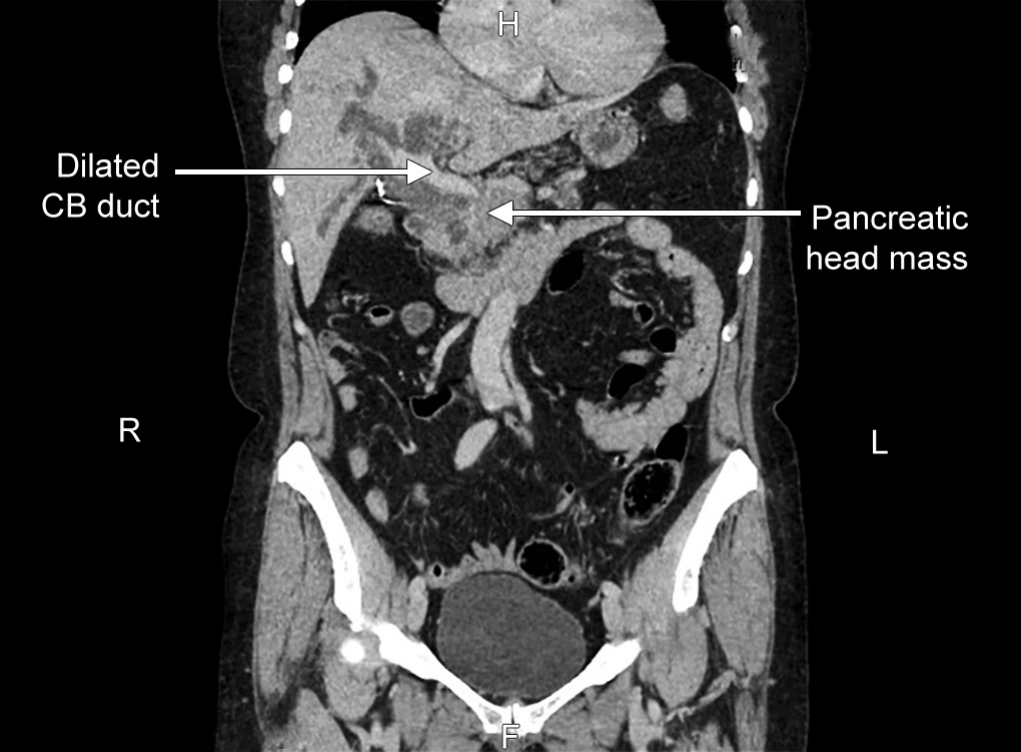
Computed tomography scan of abdomen showing pancreatic head mass
